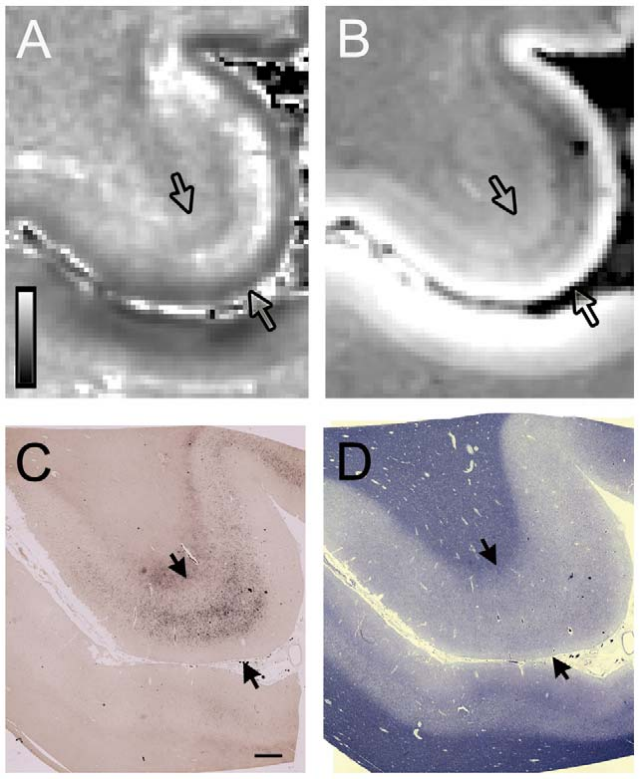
Ex vivo* 7T MRI and corresponding pathology in ALS. 7T MRI signal change corresponded to iron accumulation in deeper layers of the cortical gray in the hand knob in an ALS patient. Each panel shows an axial slice of the precentral gyrus, with the cortical gray matter boundaries indicated by arrows, and the central sulcus and postcentral gyrus below. (A) R_2_
